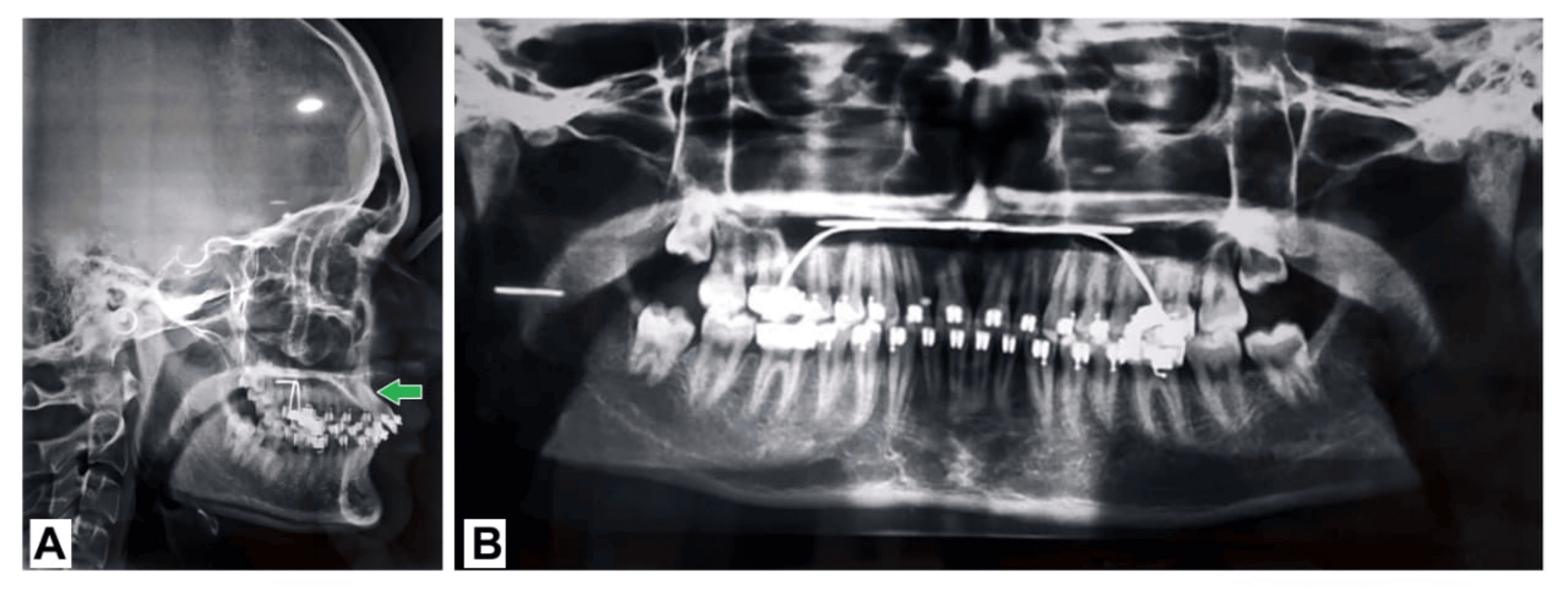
Post-treatment radiographs. (A) Lateral cephalogram: The green arrow depicts that the corrected roots of upper incisors are in cancellous bone (between palatal and buccal cortical plates) normal overjet and overbite achieved. (B) Orthopantomogram: The orthopantomogram depicts corrected crown and root angulations of upper and lower dentition.
